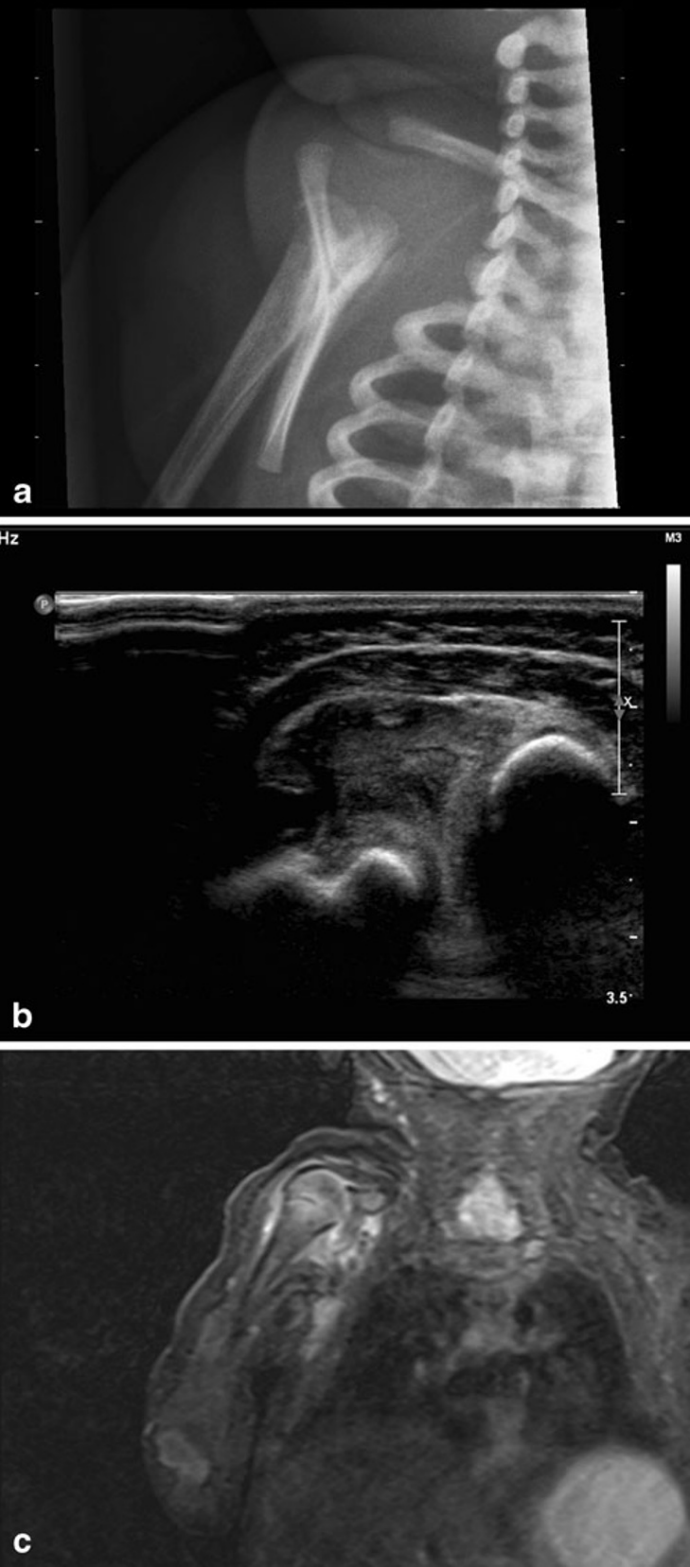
a A 1.5-month-old girl with low grade fever and reluctance to move her right shoulder. Conventional imaging shows a lucent lesion with possible cortical disruption dorsal in the head of the humerus and periostal reaction in the proximal third of the humerus. b Ultrasound study revealed hyperechoic fluid in the shoulder joint, as seen in septic arthritis. c Coronal T1 STIR series, although with motion artefact, showed high signal around the humeral head and high signal in the bone marrow of the proximal third of the shaft. The final diagnosis was septic arthritis in combination with osteomyelitis of the proximal humerus
